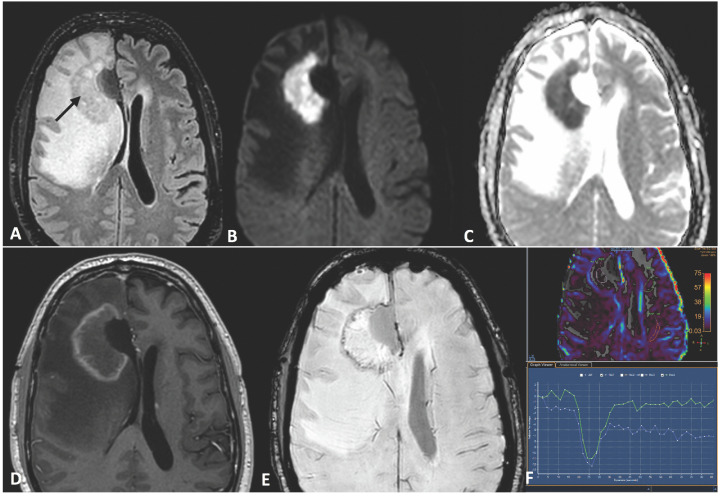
Urgent MRI in May 2023, showing an intermediate signal lesion on FLAIR imaging (black arrow in (A)) adjacent to the lateral edge of the resection cavity, with increased edema and a worsening mass effect, causing midline deviation. There is a markedly high signal intensity on diffusion-weighted imaging (DWI) (B), with very low apparent diffusion coefficient (ADC) values due to diffusion restriction (C), mild peripheral enhancement on the T1 CE sequence (D) and an irregular surrounding low signal (hemorrhage and hemoglobin degradation products) with very fine lineal hypointensities within the lesion (probably vascular structures) on susceptibility-weighted imaging (SWI) (E). On dynamic susceptibility contrast (DSC) perfusion imaging (F), relative cerebral blood volume is slightly increased (blue dynamic curve in (F)) with respect to the normal contralateral white matter (green dynamic curve in (F)).
